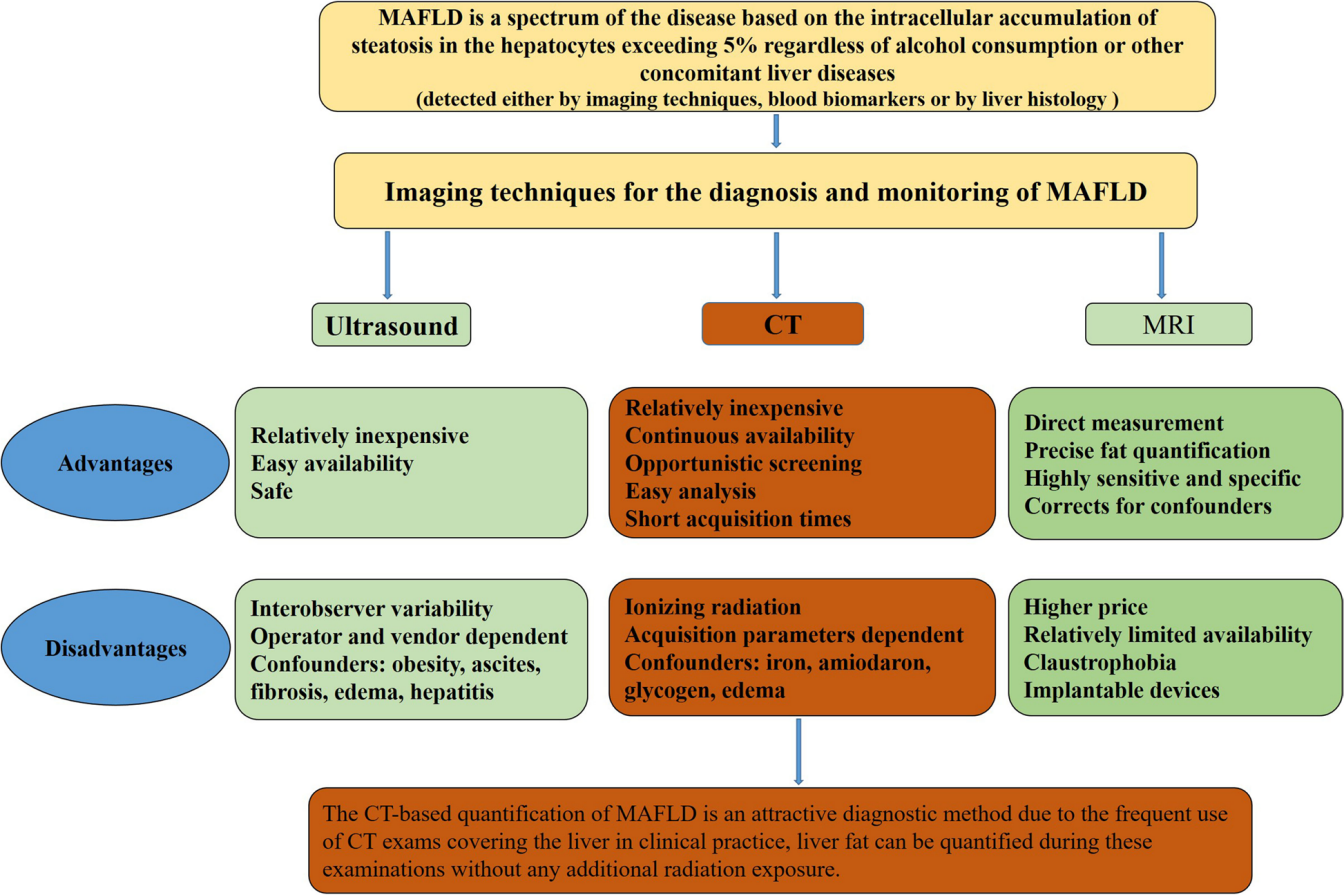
Comparison of ultrasound, CT, and MR for the diagnosis and monitoring of MAFLD. *MAFLD* Metabolic dysfunction-associated fatty liver disease, *CT* Computed tomography, *MRI* Magnetic resonance imaging, *US* Ultrasound
